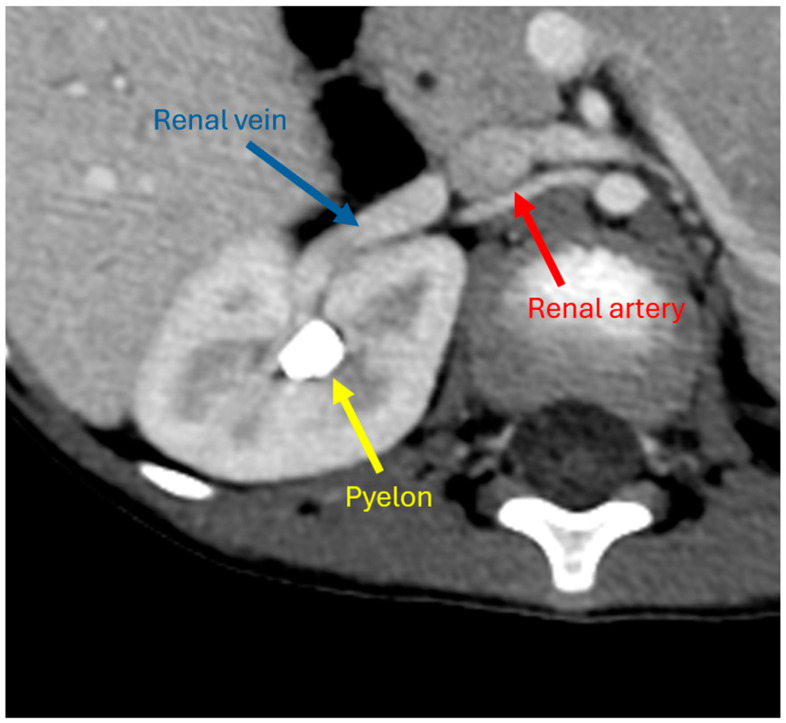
Axial contrast-enhanced CT of a 4-year-old girl with bilateral Wilms tumor obtained using a split-injection technique, achieving simultaneous opacification of the right renal artery (red), vein (blue), and ureter (yellow).
